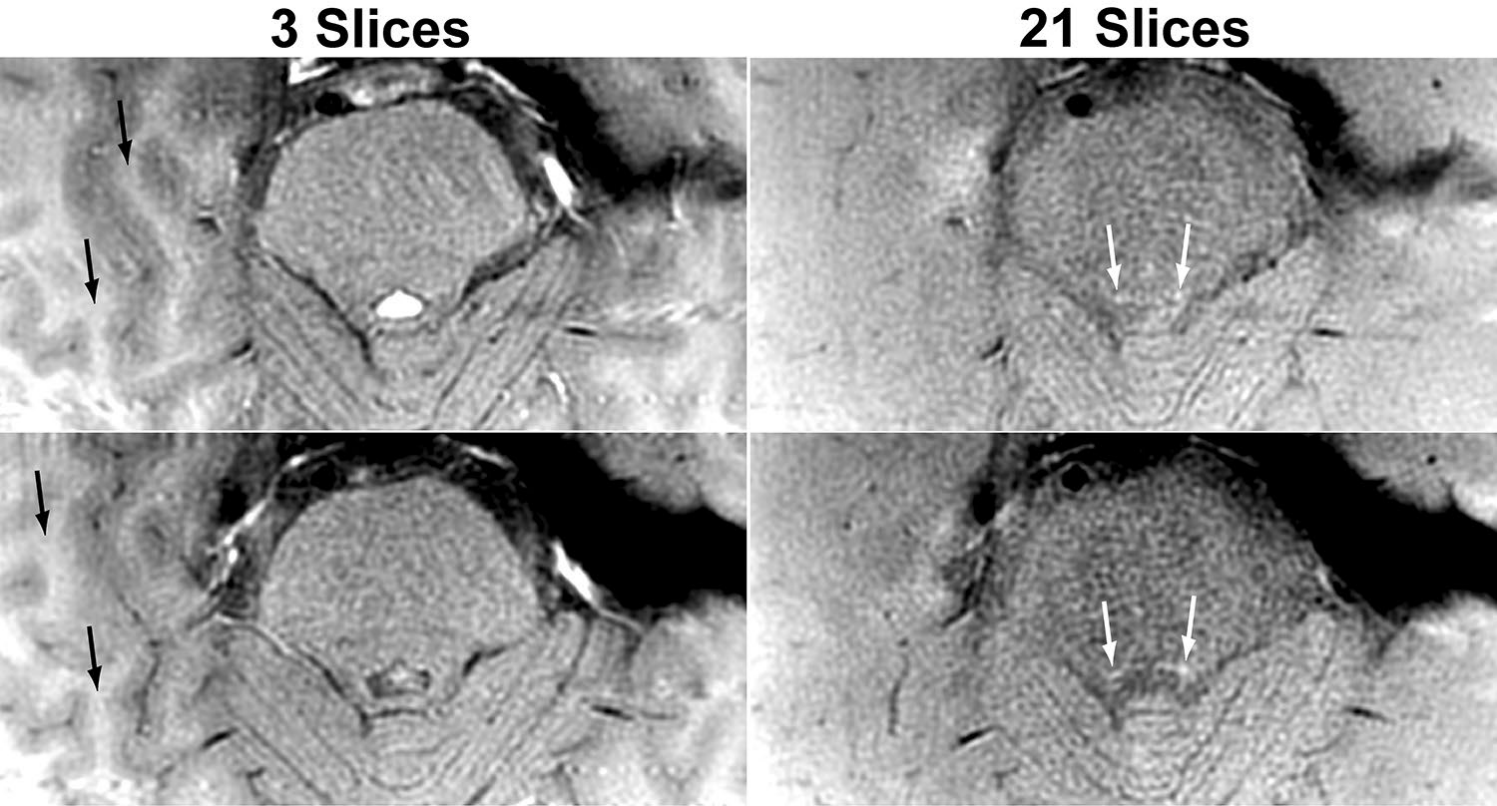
Locus coeruleus can be delineated by increasing the number of slices in a similar way as by specific off-resonance pulses. (Left column) Transversal 2D fast spin-echo MRI (TR/TE = 597/7.3 ms, flip angle = 150°) with the number of slices = 3 or (right column) 21 at two different levels of the locus coeruleus (white arrows) showing magnetization transfer effect induced by repetitive refocusing pulses. Also note that the signals of the subcortical white matter (black arrows) are predominantly suppressed by magnetization transfer effect in a 21-slice acquisition and, thus, its contrast to the cortex has disappeared or is even reversed
